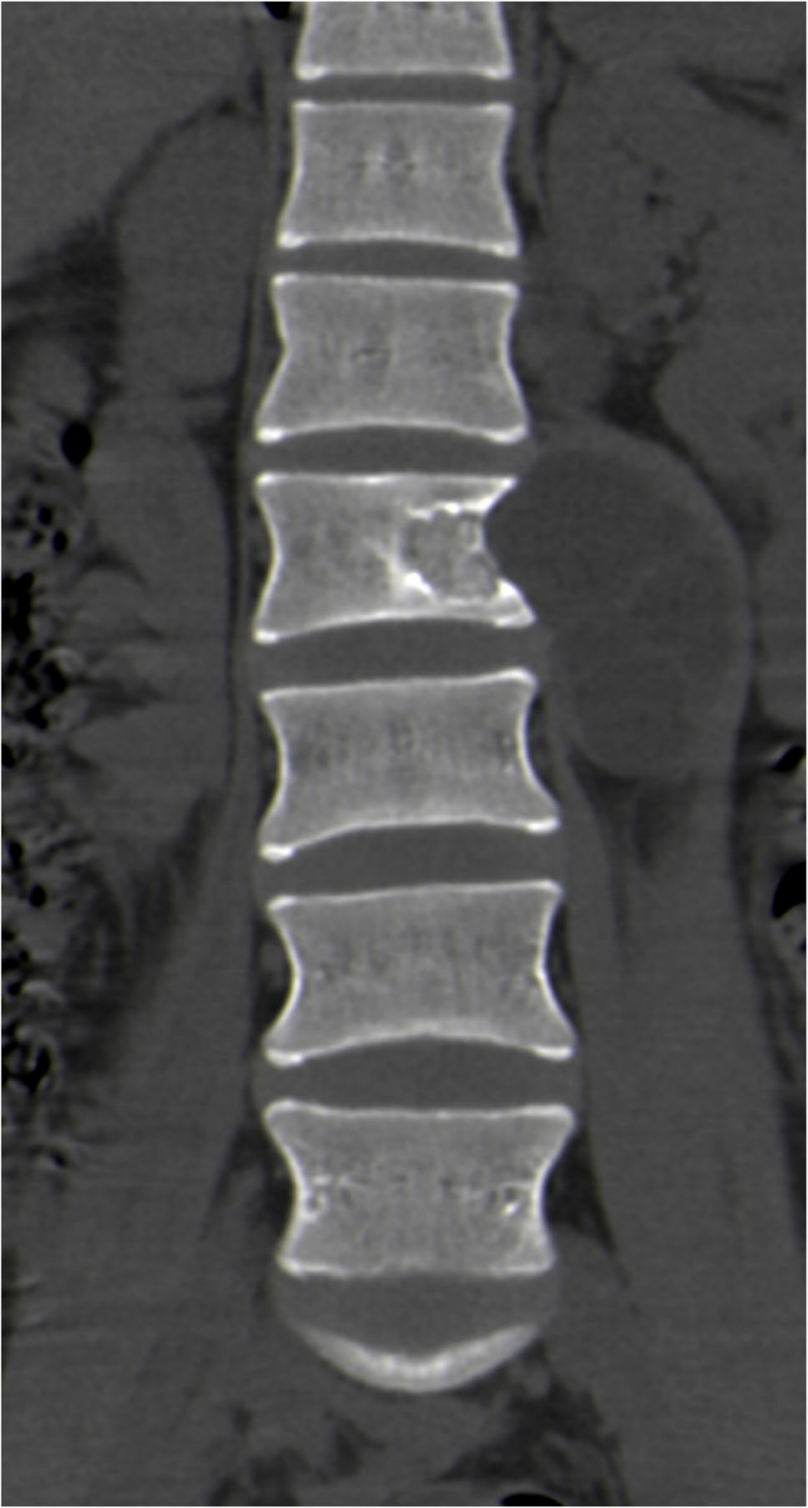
Axial CT scan revealed irregular bone destruction of the 10th thoracic vertebra and its left accessory with curved calcification of the cyst wall in a large hydatid cyst of the left soft tissue. The hydatid cyst entered the spinal canal and compressing the spinal cord
